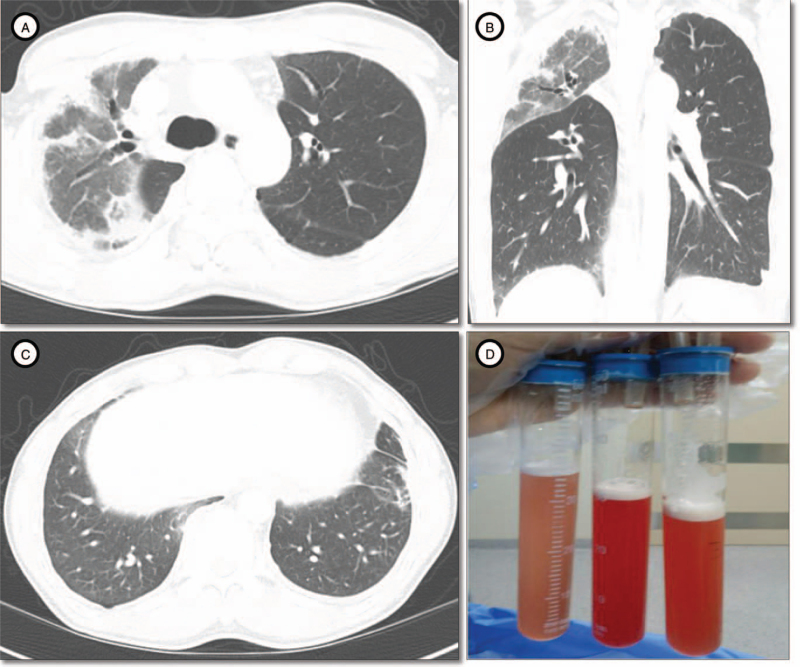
Initial computed tomographic findings and gross appearance of bronchoalveolar lavage fluid. (A, B) Computed tomography scan demonstrated consolidations, ground glass opacities, and traction bronchiectasis in the right upper lobe and the superior segment of right lower lobe. Reduced volume of the right upper lobe was noted. (C) Focal subpleural consolidation and reticular opacities were observed in the left lower lobe. (D) Bronchoalveolar lavage was performed in the anterior segmental bronchus of right upper lobe. Retrieved fluid was grossly bloody without dilution on subsequent sampling.
